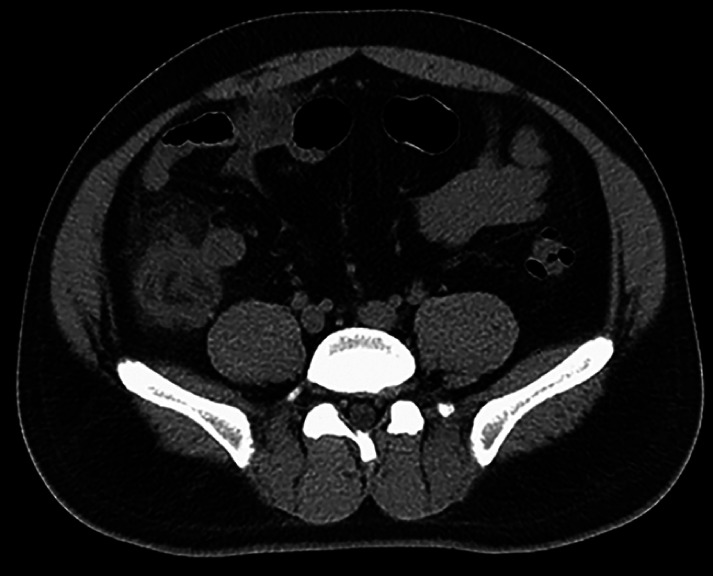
Postoperative CT demonstrated the reduction in the severity of ileocecal inflammatory exudation compared to before the procedure.
